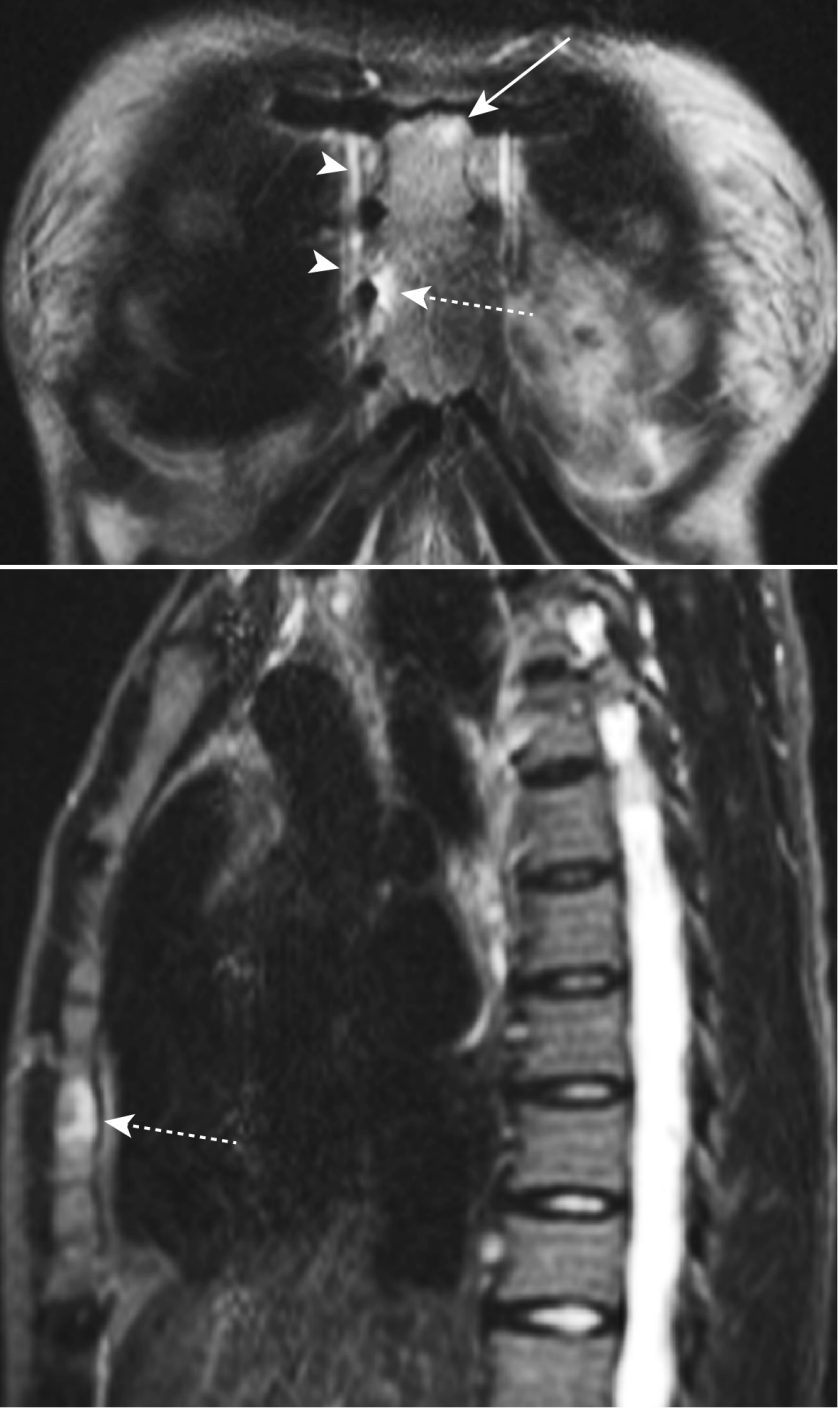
MRI inflammation of the anterior chest wall in an AS patient without clinical signs of chest wall involvement. Bone marrow edema (dashed arrows) of the fourth and fifth right sternocostal joints on frontal and sagittal STIR sequences in a 26-year-old HLA-B27-positive female patient with ankylosing spondylitis but no anterior chest wall pain. The patient reported a 2-year history of inflammatory back pain. Bone marrow edema is also seen in the lower portion of the manubriosternal joint on the left side (arrow). The mammary veins are indicated by arrowheads.
